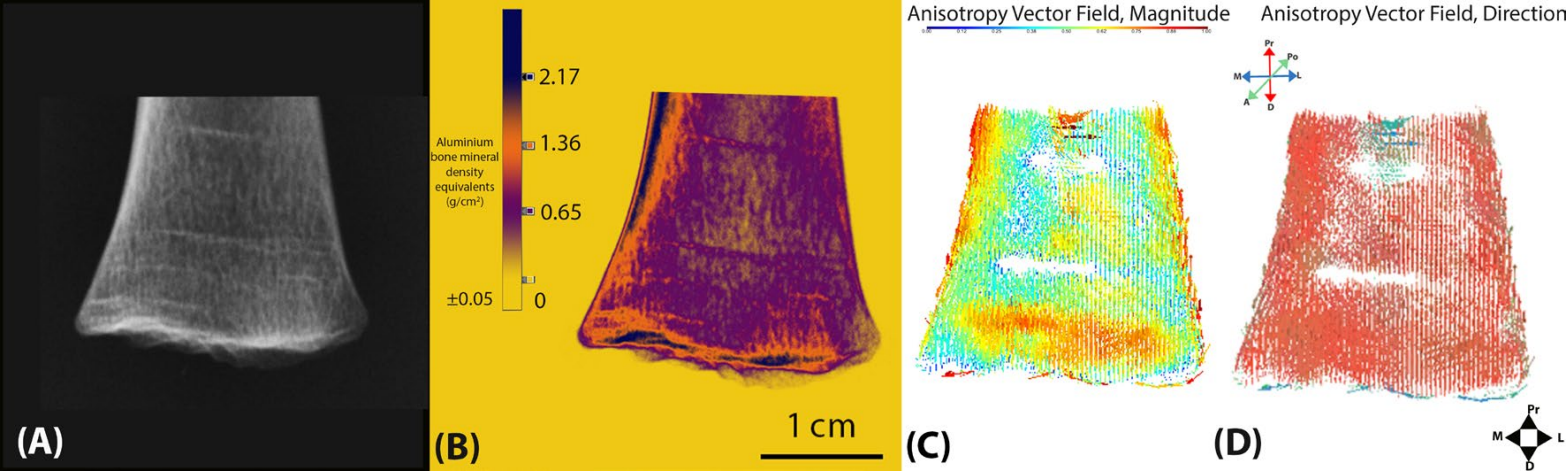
Identification of Harris lines in a left distal tibia (A) raw radiograph, (B) radiographic color map from Reid, Davies, and Cunningham (2023), (C) anisotropy vector field, magnitude, and (D) anisotropy vector field, direction. Sparse areas in vector fields coincide with Harris lines in radiographs.
